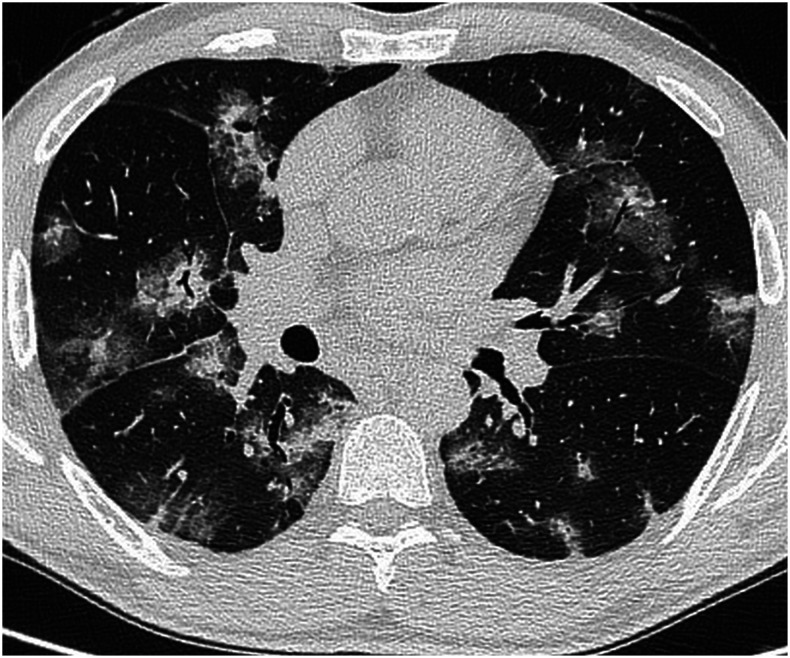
Chest computed tomography showing bilateral nodules and consolidations, surrounded by ground-glass opacities, and resulting in a halo sign, with prevalent peribronchovascular distribution. Air bronchograms are bilaterally recognizable.
